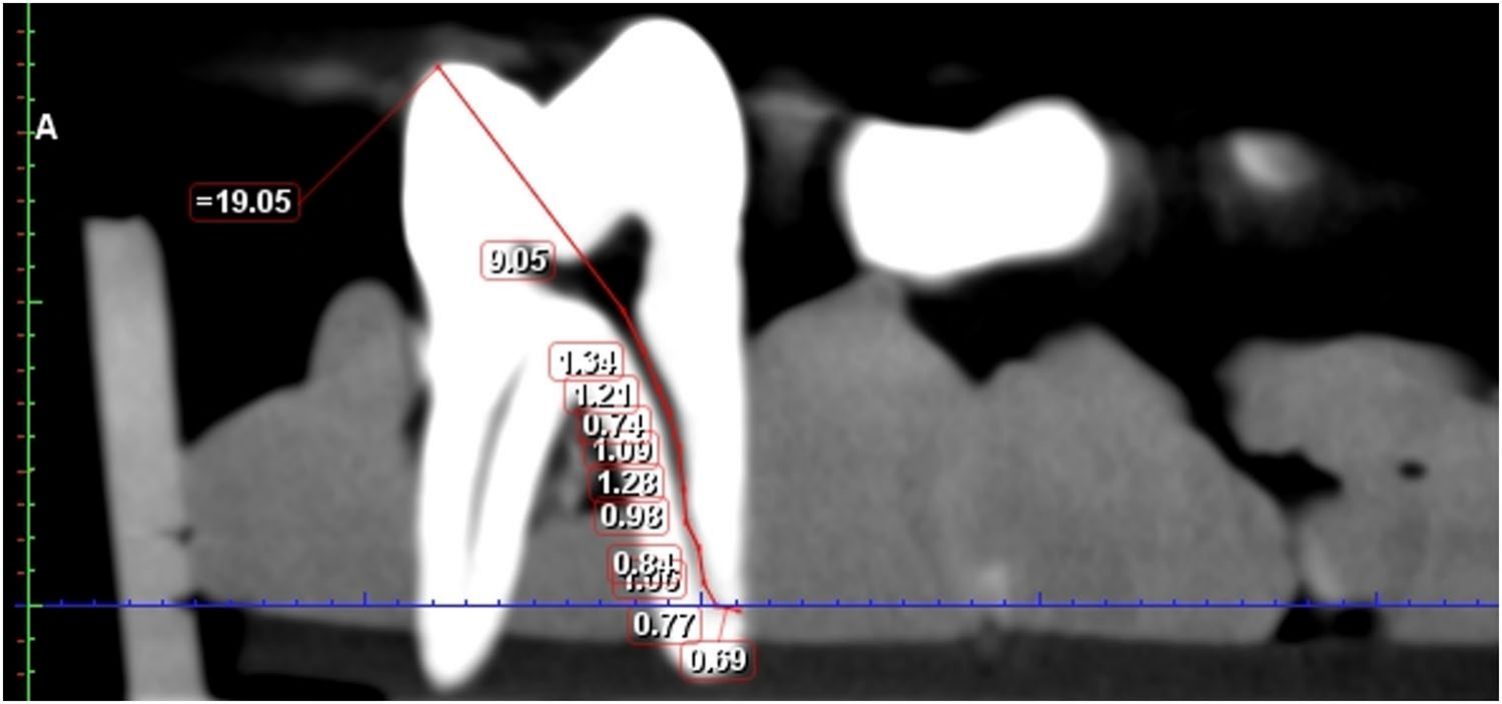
CBCT measurements of root canal length at slice thickness of 1.2 mm.
